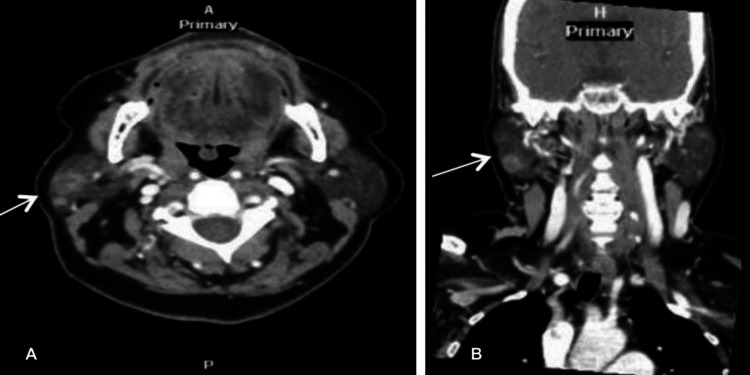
CT neck imaging of the parotid gland lesion. Axial (A) and coronal (B) views of a contrast-enhanced CT neck scan revealing a well-defined, non-homogeneous lesion in the right parotid gland (white arrows) measuring approximately 13 x 12 x 10.5 mm. No surrounding tissue infiltration is observed
